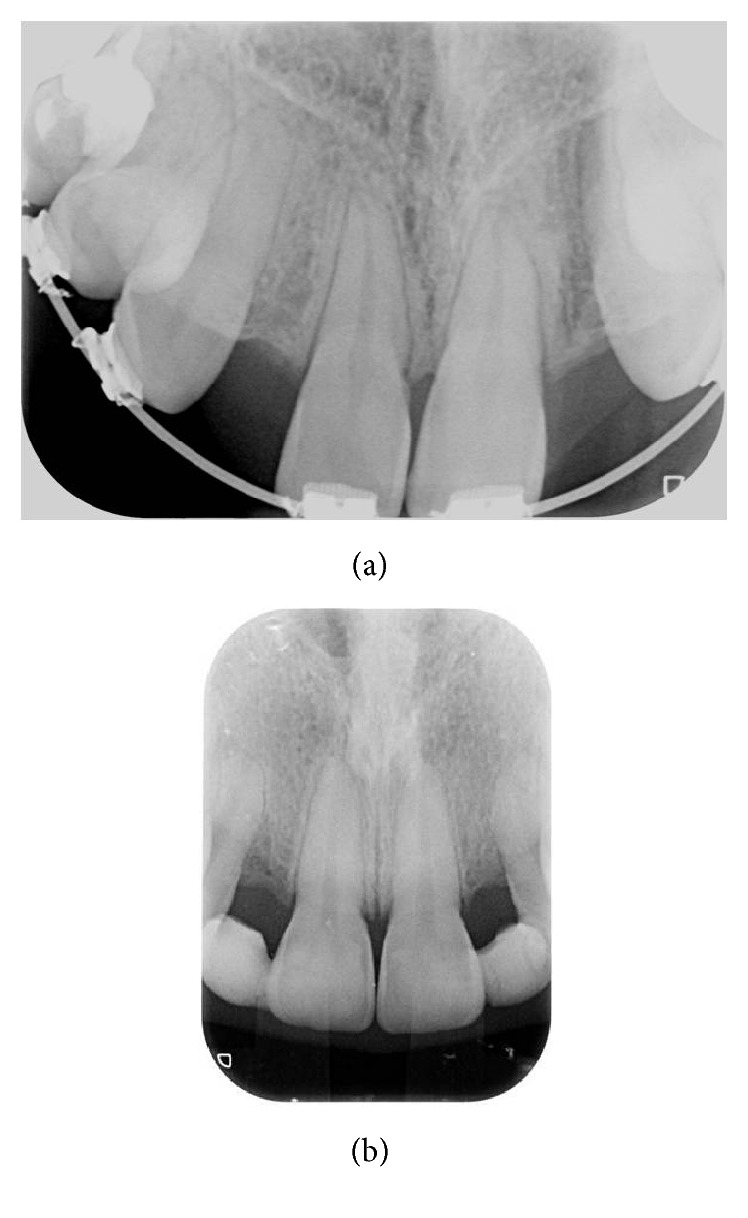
Radiographic preoperative condition.
